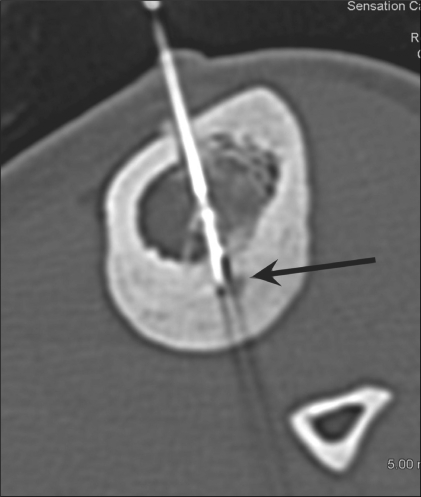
Osteoid osteoma of the tibia. CT scan in the supine position shows an approach to the lesion in the posterior cortex, through the nonaffected anterior cortex (arrow)
